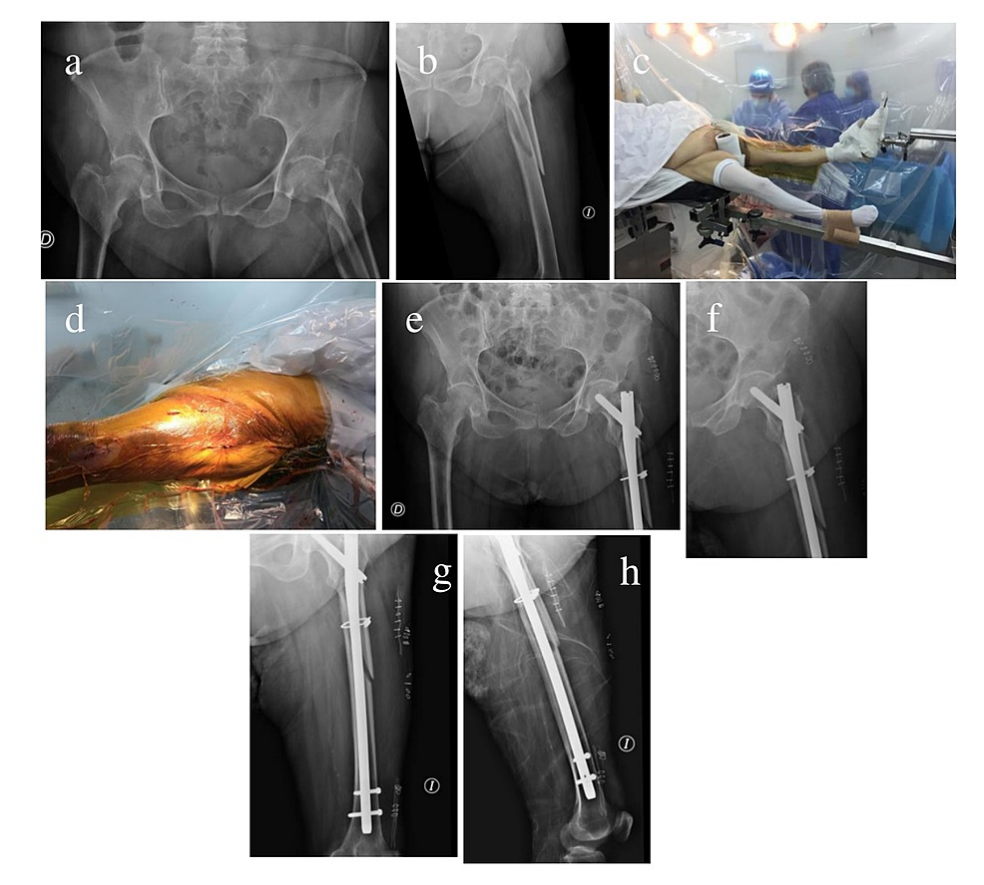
Left subtrochanteric fracture and well leg scissor position (a and b) A 75-year-old female patient; preoperative anteroposterior (AP) pelvis and femur X-rays demonstrating a left subtrochanteric fracture. (c) Well leg scissor position and a transparent vertical field. (d) Transparent vertical field on the surgeon’s side showing mini incisions in the operated femur. (e-h) Immediate postoperative pelvis and femur X-rays in the AP and lateral views.
